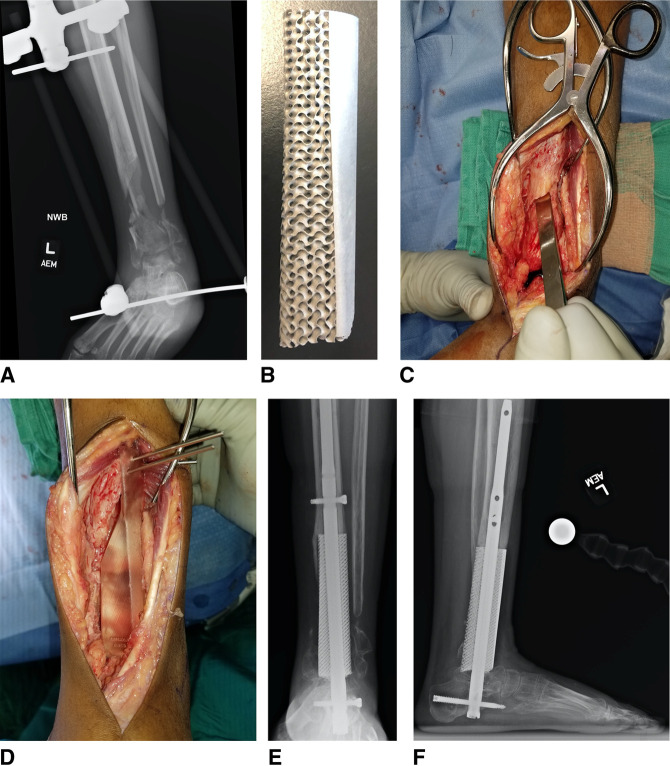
A, Anterior-posterior (AP) radiograph of the left leg of a 22-year-old woman who was injured in a motor vehicle collision. She sustained an open tibia and fibula fracture. Bone was lost at the scene, leaving a large bony defect. B, Custom three-dimensional (3D) printed implant designed to fill the bony defect. C, Intraoperative image showing the distal tibia fracture and bone loss. D, Three-dimensional printed anatomic spacer block to assess alignment and length and to perform intramedullary reaming. E, AP radiograph demonstrating successful implantation of the 3D-printed implant. F, Lateral radiograph demonstrating successful implantation of the 3D-printed implant.
